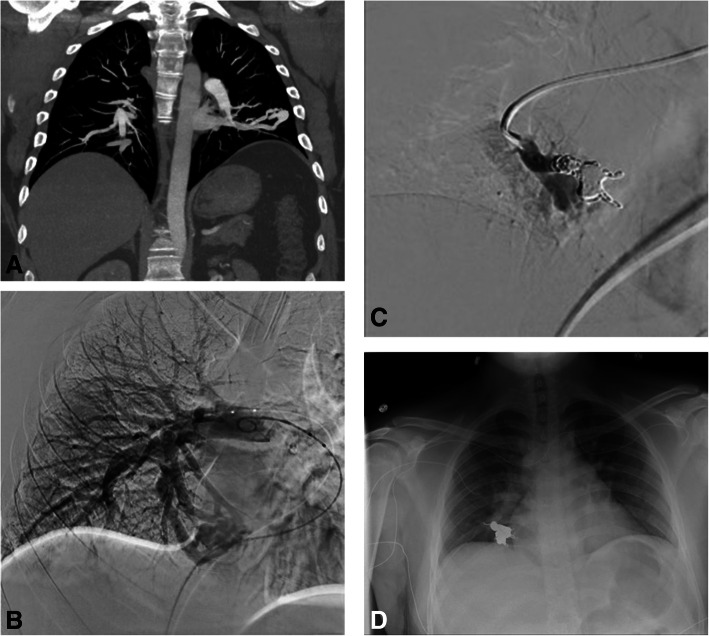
A Coronal CT with contrast shown in a maximum intensity projection demonstrates a simple pulmonary AVM in the left lower lobe (arrow). B Digital subtraction angiography demonstrating a complex pulmonary AVM in the right middle lobe with multiple feeding subsegmental pulmonary arteries. C Digital subtraction angiography post coil embolization of the complex right middle lobe pulmonary AVM demonstrating no flow through the PAVM. D Follow-up chest x-ray in the same patient demonstrating the coil pack in the embolized right middle lobe PAVM
